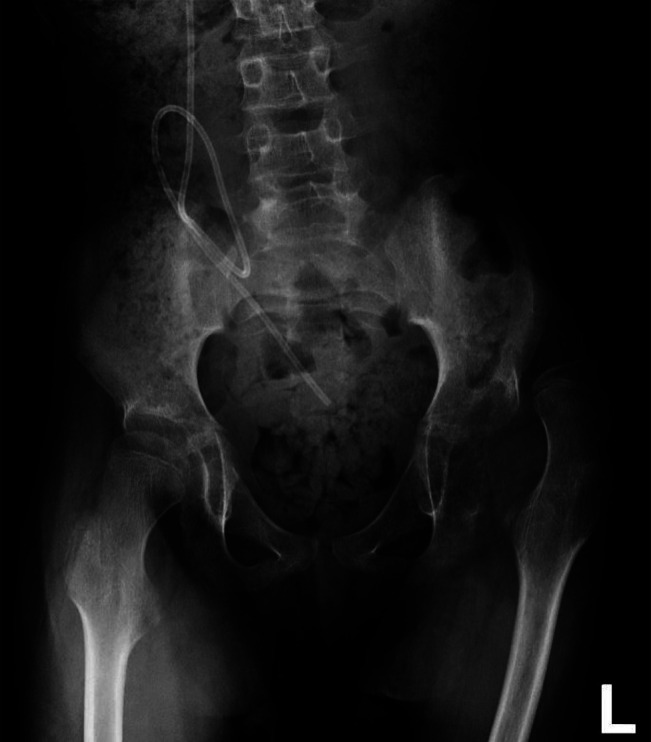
Six-week postoperative anterior-posterior radiograph after the second surgery of the pelvis revealing a left hip dislocation.
